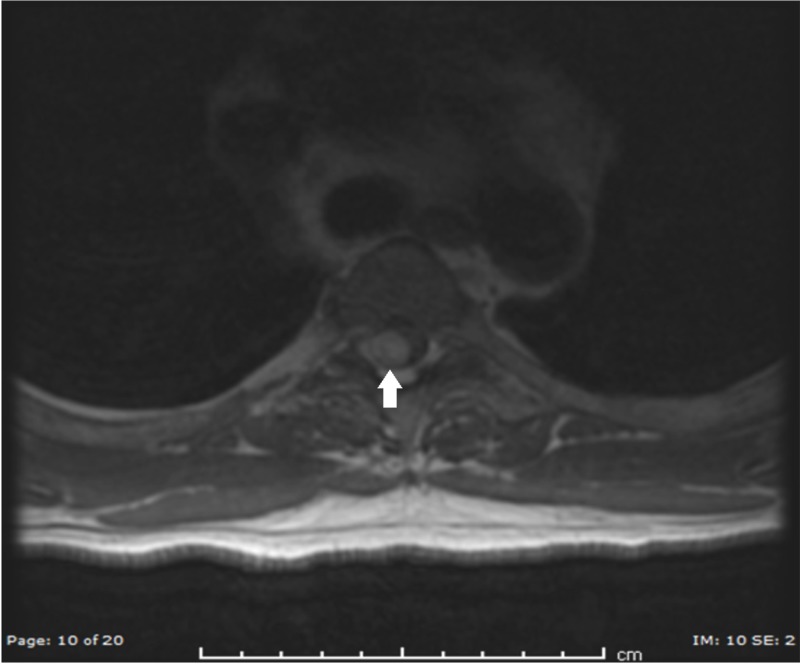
Preoperative T2 axial MRI showing a hyperintense lesion at the T3-T4 level MRI: magnetic resonance imaging
